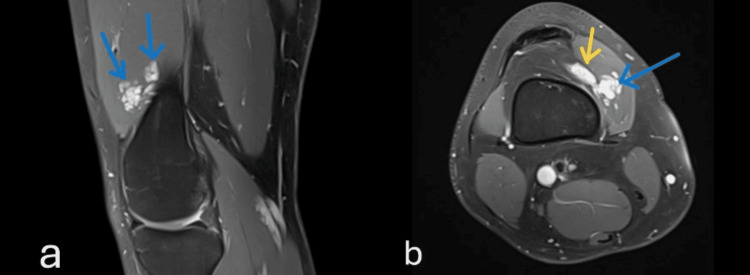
MRI of the right knee a) Proton Density Fat-Saturated sagittal sections imaging and b) Proton Density Fat-Saturated axial sections imaging showing well-defined, non-homogeneous, hypertension signals of the hemangioma (intramuscular mass with blue arrow and intrasynovial mass with yellow arrow)
